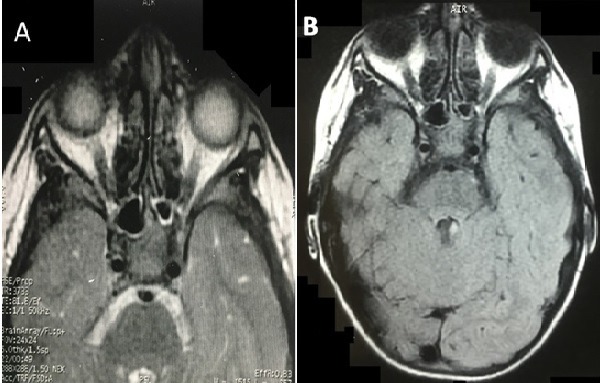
Postoperative brain and orbital MRI, axial T2 weighted sequence (A) and FLAIR (B) showing the total disappearance of the cyst with optic nerve decompression
